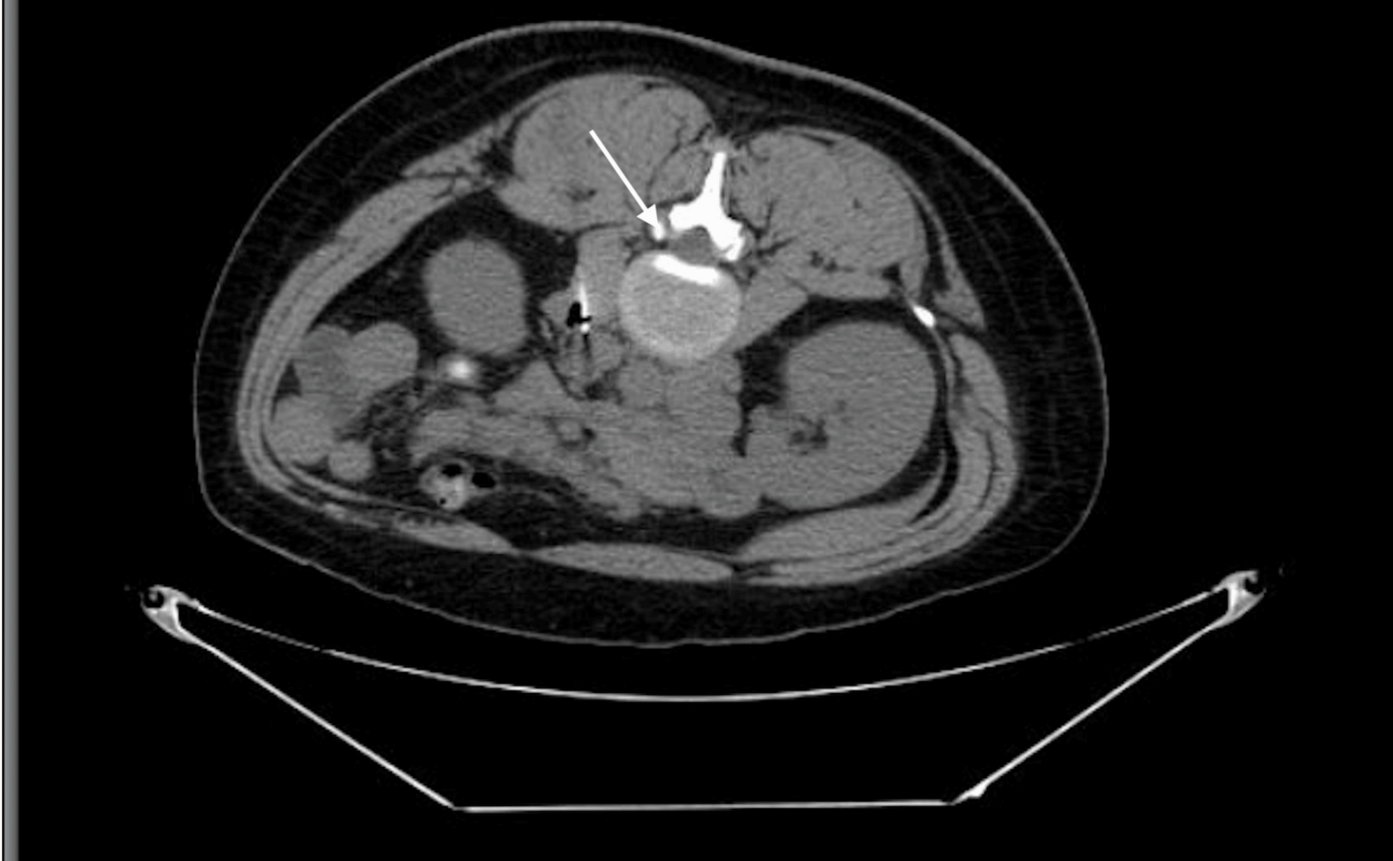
Axial computed tomography image of the abdomen CT = computed tomography. Axial imaging demonstrates a discrete left para-aortic retroperitoneal lymph node (arrow) measuring approximately 2.1 cm, corresponding to findings described in the case presentation.
